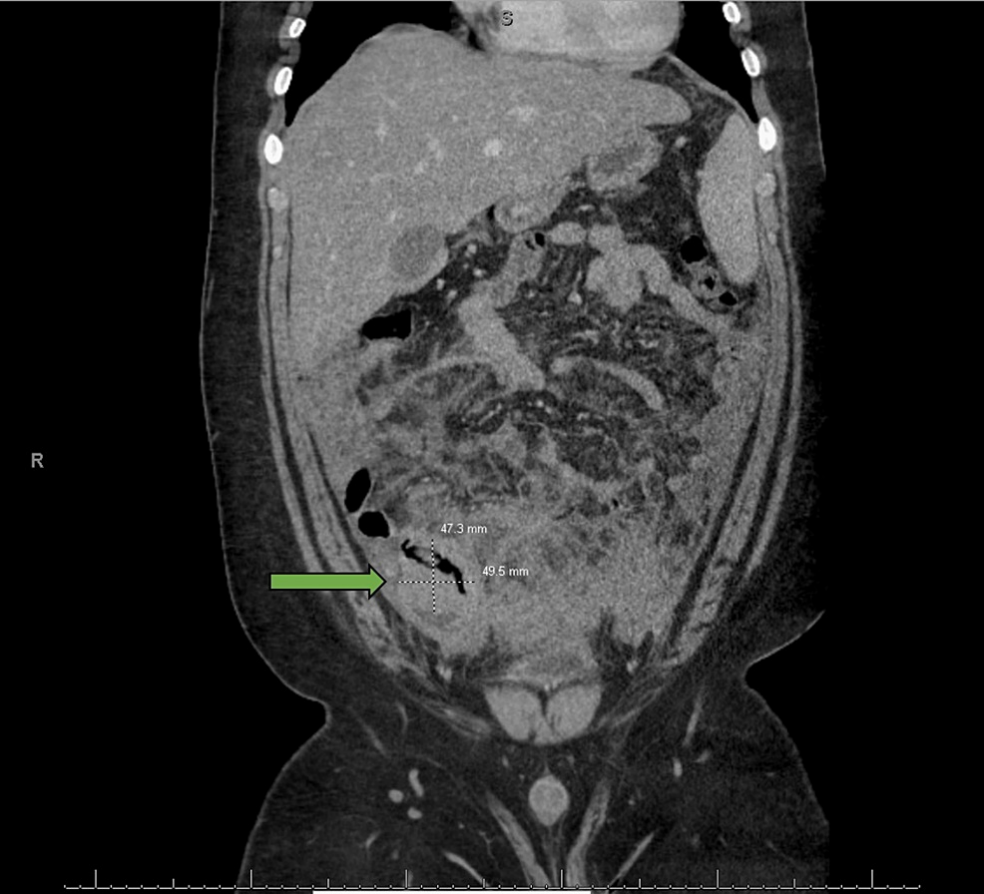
Computerized tomography (CT) scan of the abdomen CT scan of the abdomen showed extensive inflammatory stranding in the lower abdomen and pelvis centered in the right lower quadrant and a thick-walled fluid collection (as indicated by the arrow) in the pelvis concerning for ruptured appendicitis.
